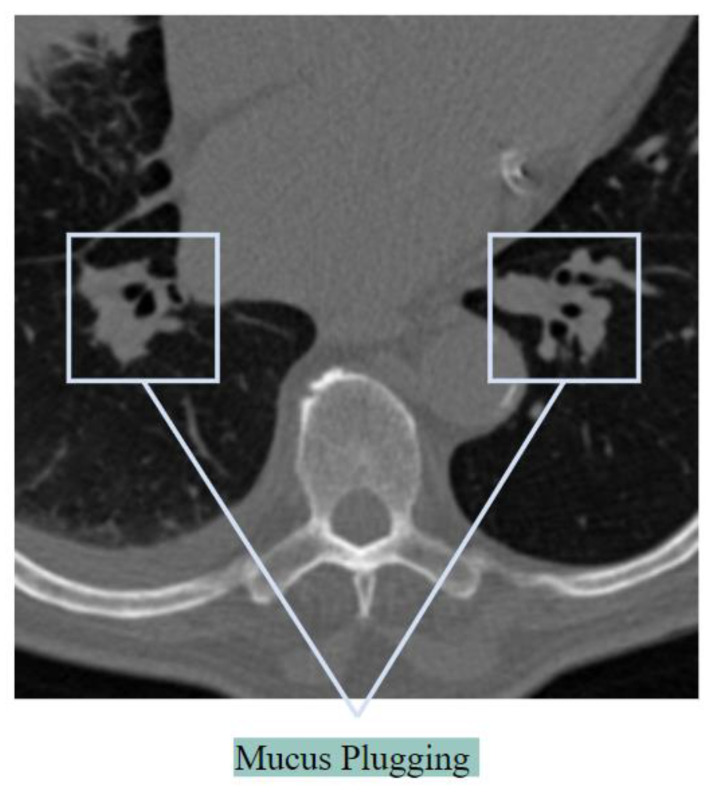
Mucus plugging as seen in a CT scan.
